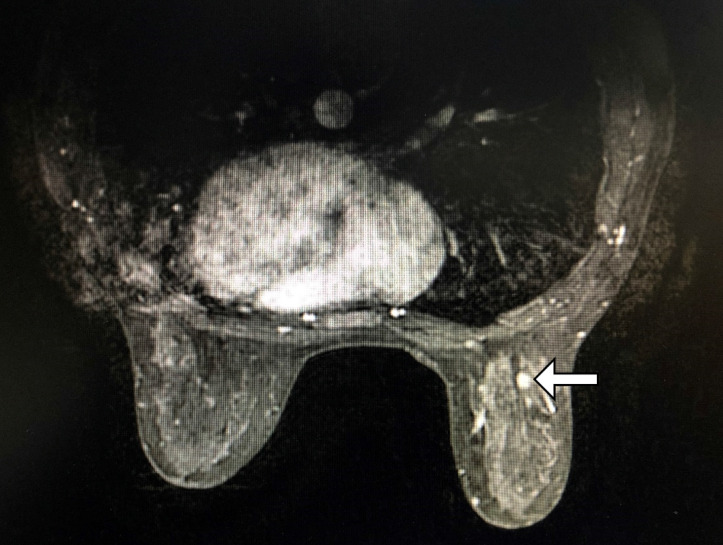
Examples of MRI-detected single right breast lesion of a 47-year-old woman (white horizontal arrow), which is invisible on the corresponding ultrasound and mammography, and the radiologist's report shows BI-RADS 4. This lesion can't be seen on ultrasound after re-identification and then MRI-guided localization is performed with a wire. Pathology after surgery revealed medium nuclear grade ductal carcinoma *in-situ*.
